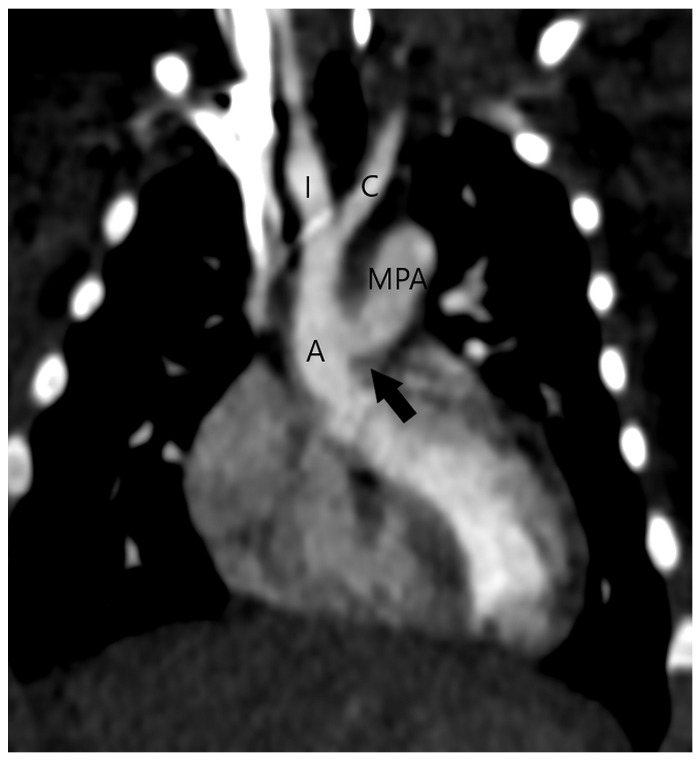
Type B interrupted aortic arch in a patient with aortopulmonary septal defect type 2 (arrow); multiplanar reconstruction of CT angiography. The aorta (A) ends with bifurcation on the innominate artery (brachiocephalic trunk, I) and the left common carotid artery (C). Main pulmonary artery (MPA).
